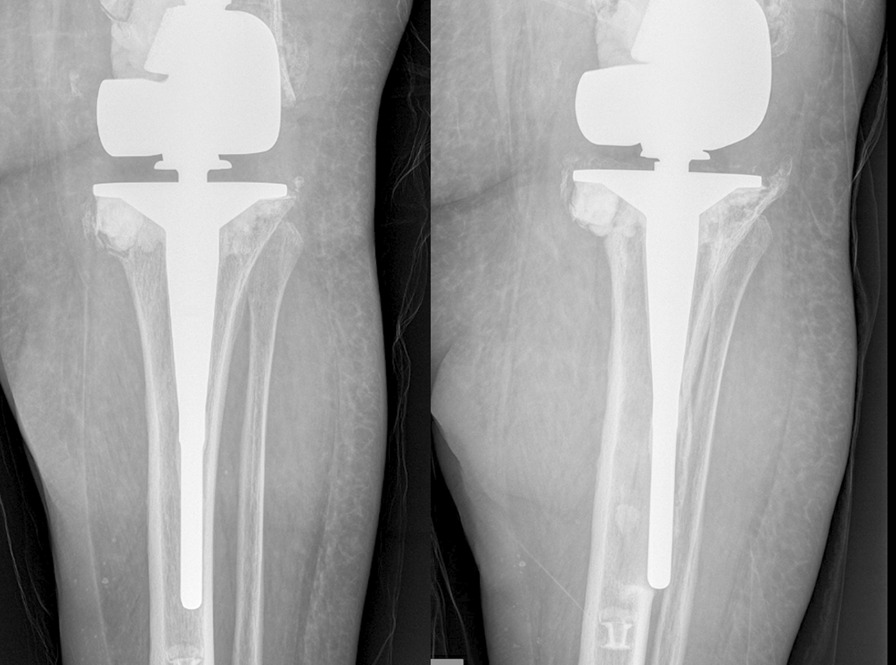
X-rays left knee. One year progression of failed left knee tibial component with chronic penetration of the lateral tibial cortex evident by neocortex and reactive changes
